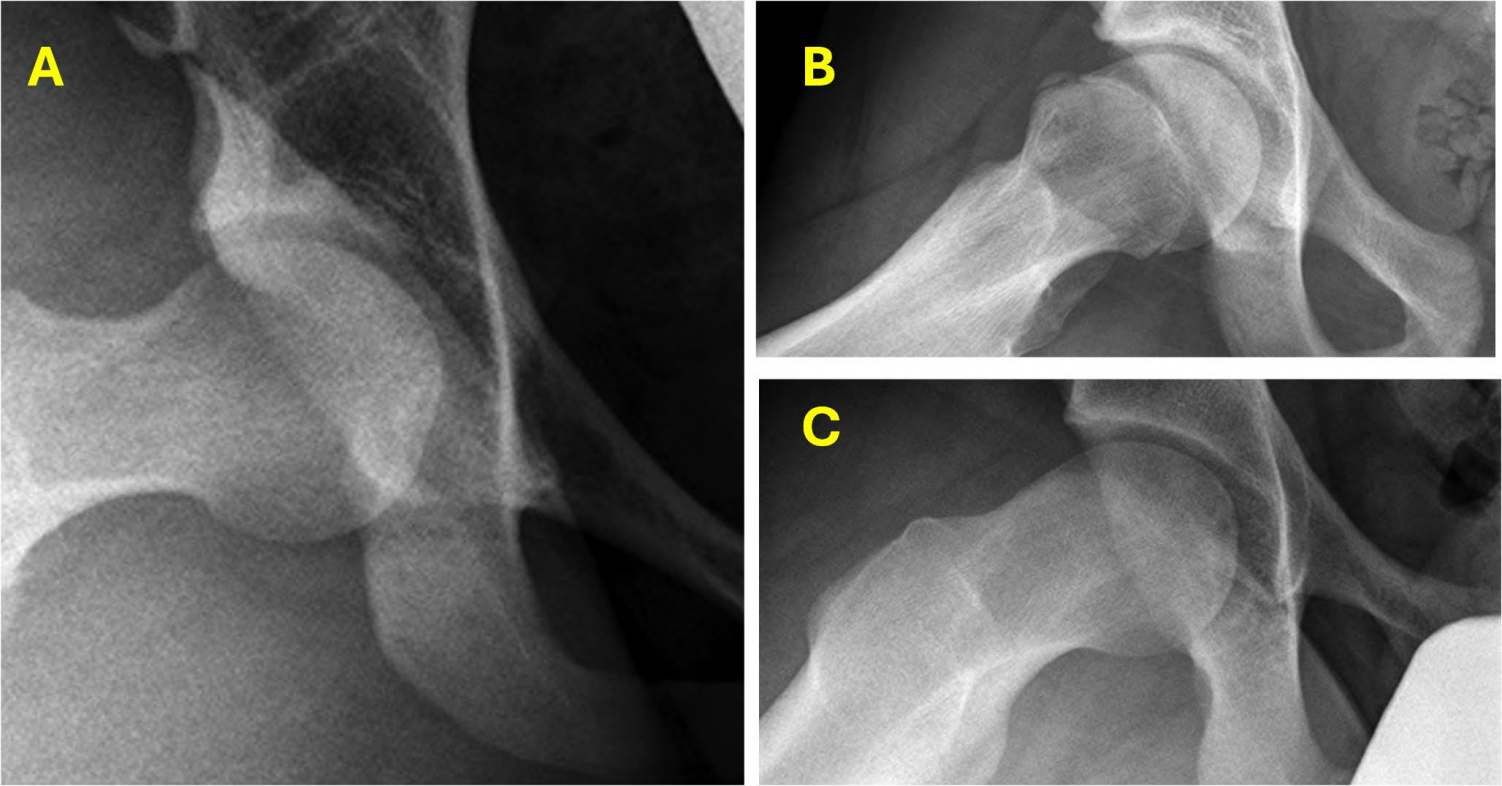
The radiographic images demonstrate three distinct hip presentations. (A) normal hip morphology without symptoms, (B) a symptomatic hip displaying noninflammatory cam changes, and (C) a hip exhibiting the characteristic inflammatory beak formation of the cam deformity, which is frequently observed in patients with underlying inflammatory conditions [12]
